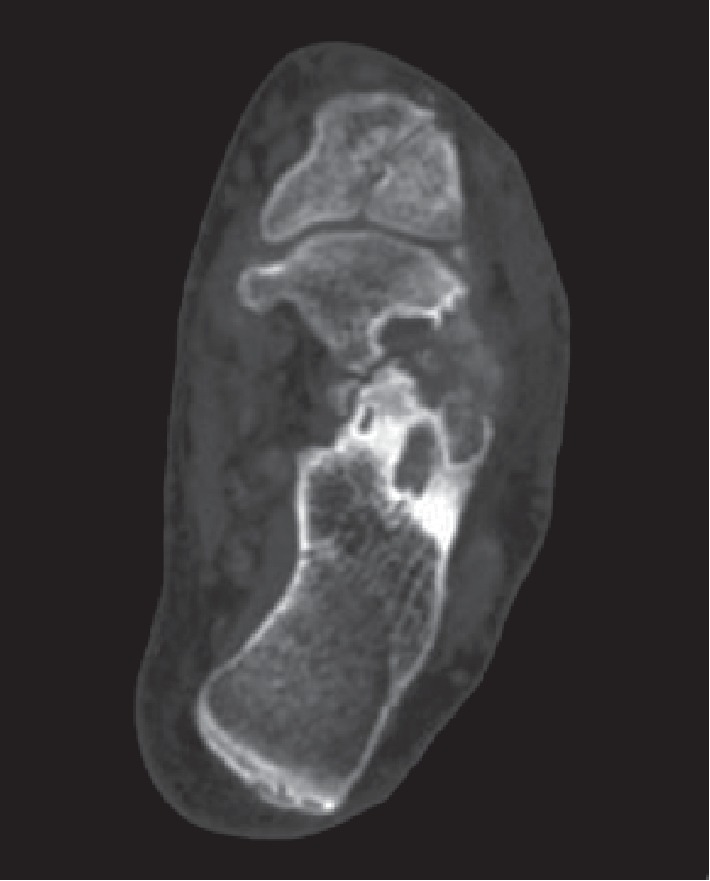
Erosion and intra-articular swelling with tophaceous masses (CT of the left foot).
